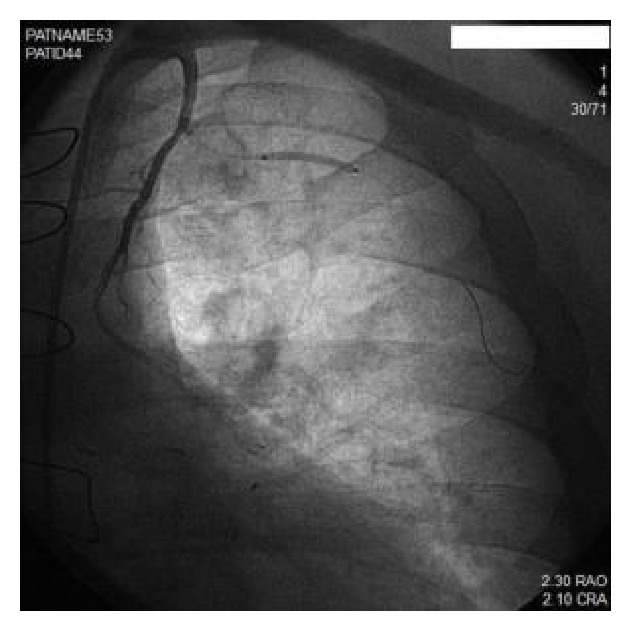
The LIMA was engaged with a 6-French IMA guide catheter. The Doppler wire was then advanced into the mid-portion of the LIMA distal to the first thoracic branch collateral with balloon occlusion of side branch.
